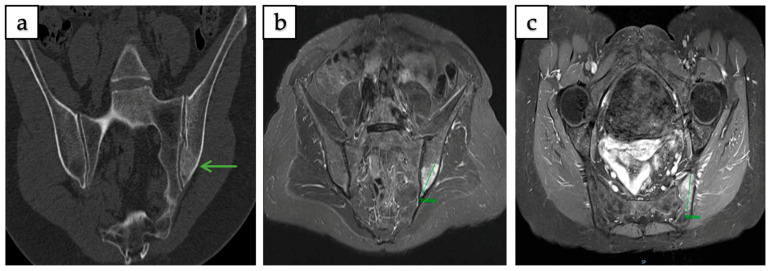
Intraosseous hibernoma of the left iliac bone confirmed by biopsy in a 63-year-old woman, measuring 38 mm in its largest axis. (a) Coronal CT scan showing an ill-defined, mildly sclerotic lesion (green arrow). (b,c) T1-weighted fat-saturated post-gadolinium MRI in coronal and axial planes demonstrating a heterogeneous lesion with contrast enhancement.
